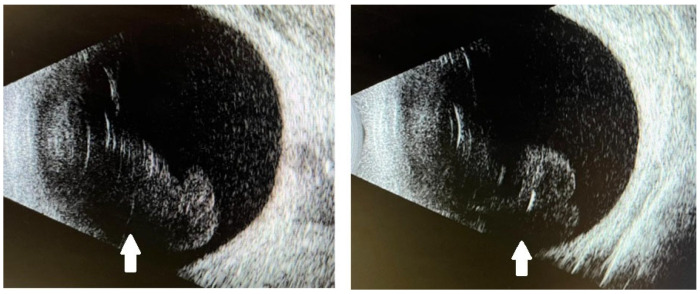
B-scan ultrasonography of both eyes showing attached retinas and symmetrical vitreous opacities (white arrows) consistent with intraocular inflammation.
